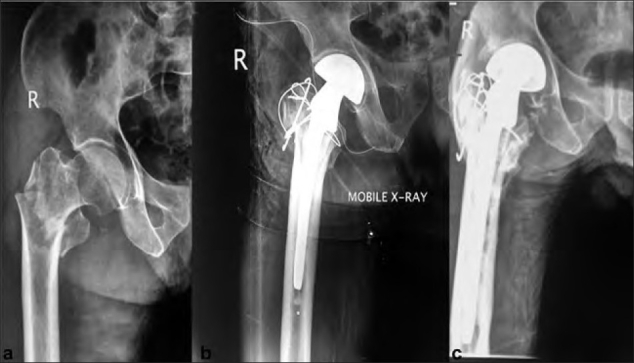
X-ray right hip joint anteroposterior view (a) 80-year-old male showing an unstable intertrochanteric fracture, (b) treated with primary partial hemiarthroplasty, (immediate postoperative) (c) 2-year follow-up radiographs
